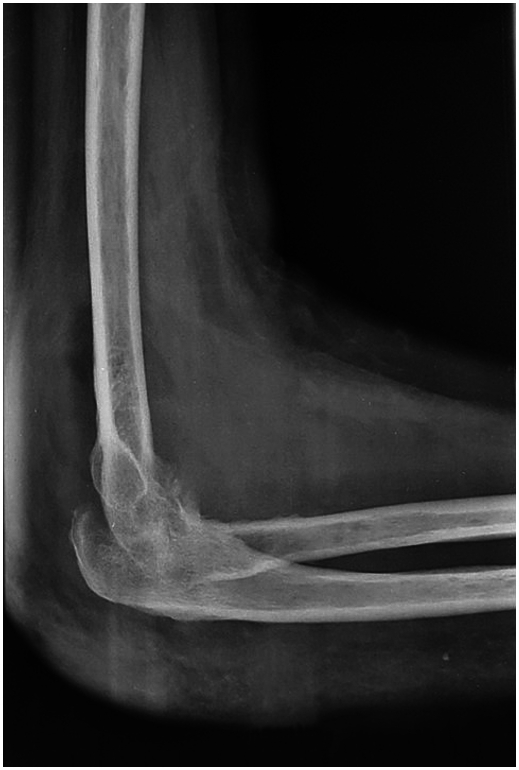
Lateral plain radiograph of the right elbow demonstrating marginal periarticular osteolysis and elbow subluxation.
